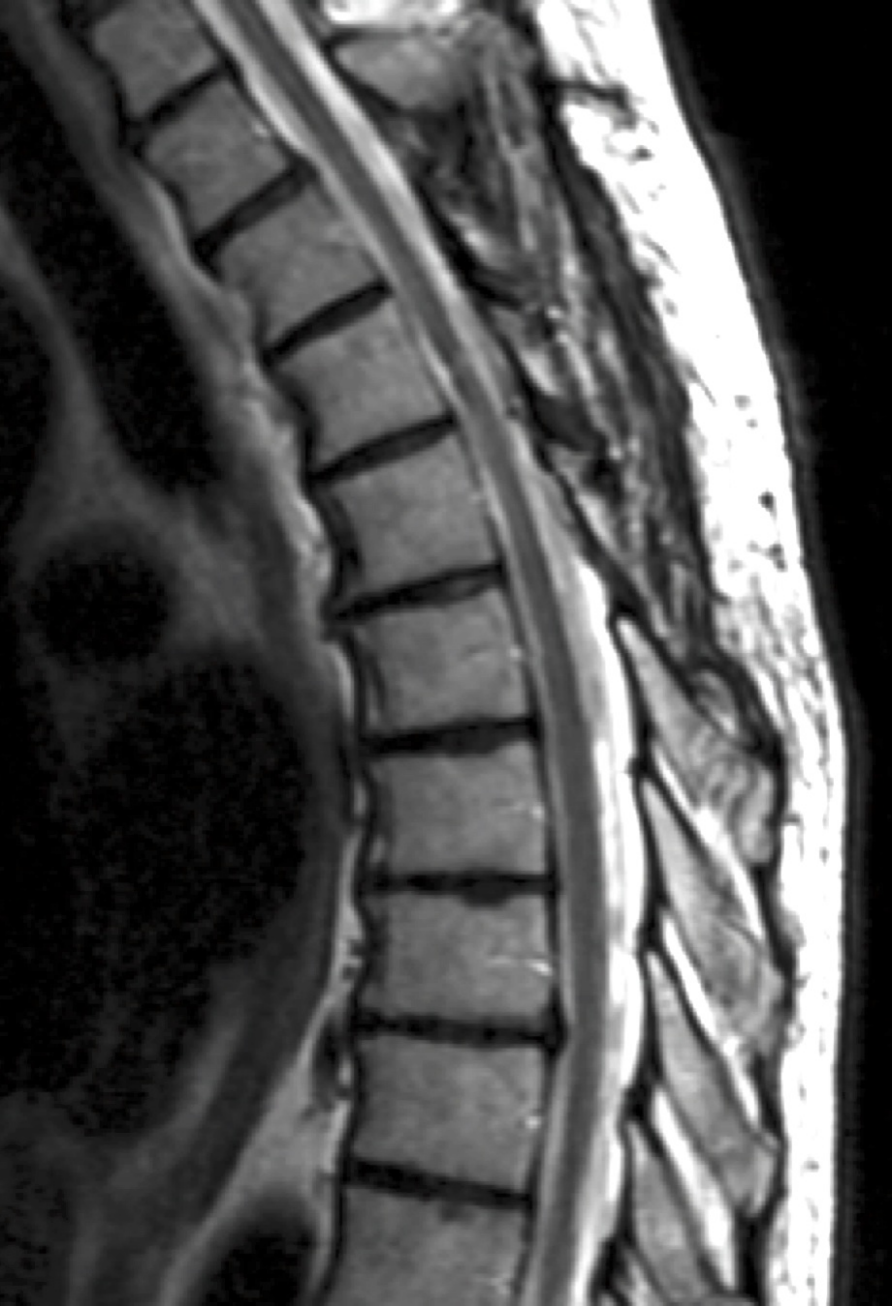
Thoracic spine MRI demonstrating no residual intramedullary cavernous malformation at the 10-month follow-up.
